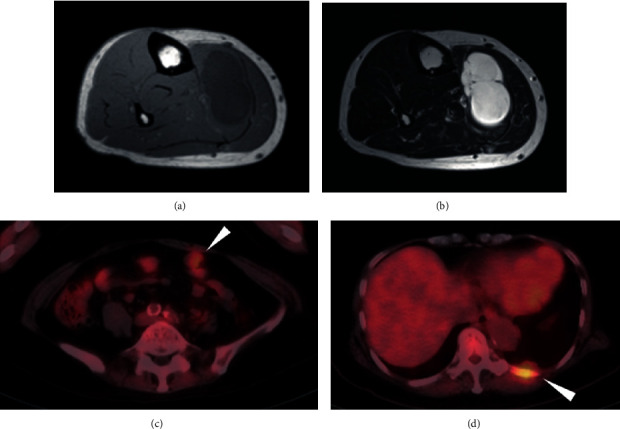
Metastatic tumors in the lower right leg, abdominal space, and chest wall. (a) T1-weighted and (b) T2-weighted MRI showing metastatic tumor in the lower right leg. Metastasis in (c) abdominal space and (d) chest wall in PET.
